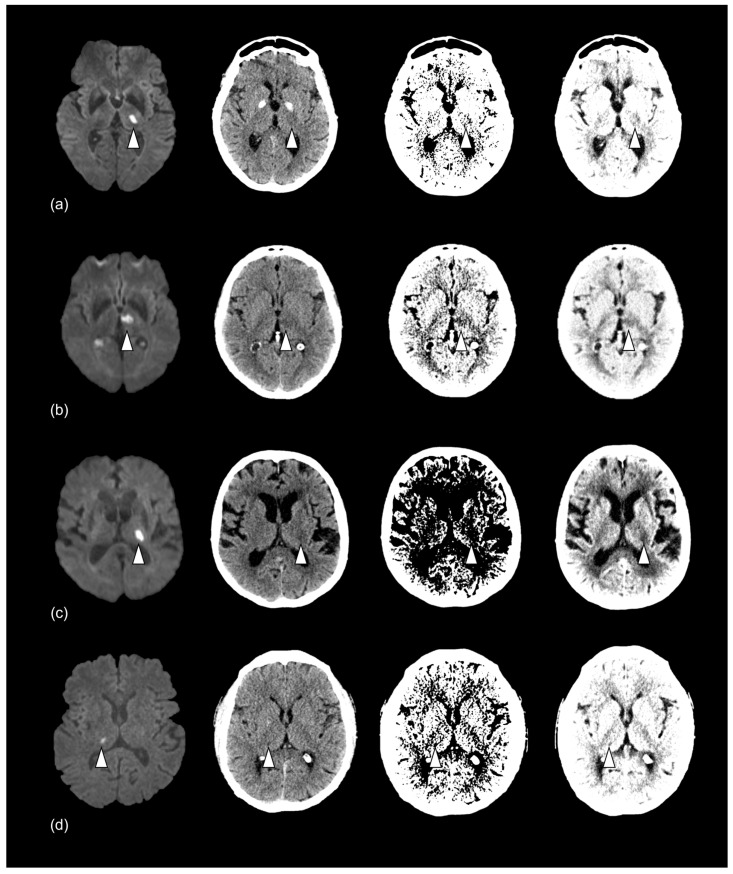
Imaging of thalamic RSSI for the time windows of (a) 0–6 h, (b) >6–12 h, (c) >12–24 h, and (d) >24–36 h. Confirmed infarct (white arrowhead) on DW-MRI (left) and corresponding unprocessed, window-, and BC-optimized NCCT (right), respectively. The NWU is 6.2% (a), 9.2% (b), 15.6% (c), and 24.3% (d). The infarct was not detected in any NCCT (a), detected in window- and BC-optimized NCCT (b), only detected in BC-optimized NCCT (c), and detected in all NCCT (d). RSSI = recent small subcortical infarct, DW-MRI = diffusion-weighted magnetic resonance imaging, BC = Best Contrast, NCCT = non-contrast computed tomography. NWU = net water uptake.
